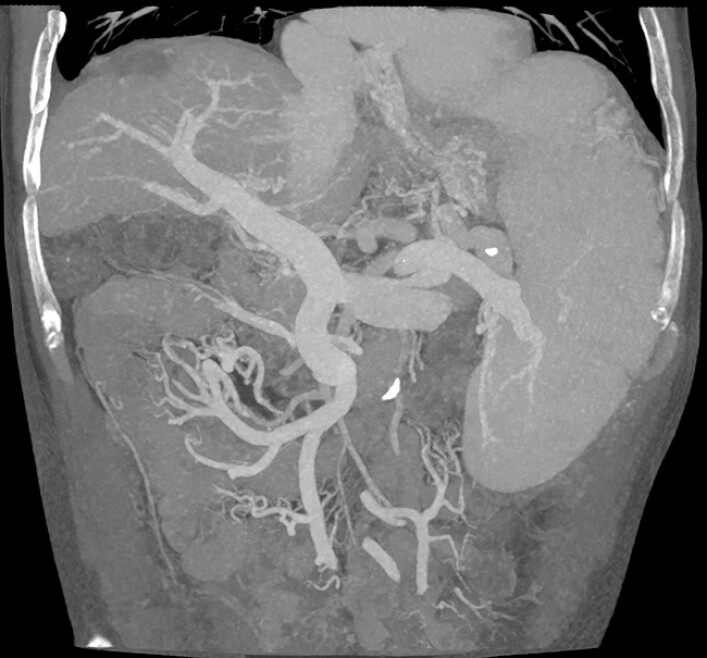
CT venography showed opacification of the main portal vein and right branch. CT, computed tomography.
